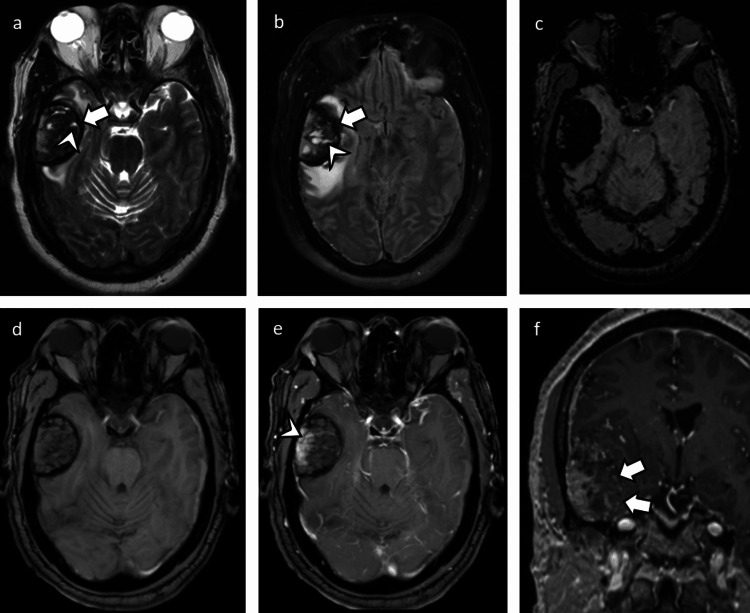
MRI brain without and with contrast a and b: T2 and FLAIR sequences demonstrating a lesion with a T2 hypointense rim (arrows) with surrounding edema. Internal fluid-fluid levels likely related to the internal hemorrhagic component. Additional regions of hemorrhage with fluid-fluid levels (arrowheads) are seen outside of the T2 hypointense rim. c: SWI sequence demonstrating marked susceptibility artifact representative of the hemorrhage, which appears to coincide with the regions that exhibit fluid-fluid levels. d and e: T1 precontrast and postcontrast imaging demonstrate an enhancing component of tumor (arrowhead) along the lateral margin. f: Zoomed-in coronal T1 postcontrast demonstrating enhancing component of the tumor along the lateral margin with the additional suggestion of thin peripheral enhancement (arrows) MRI: magnetic resonance imaging; FLAIR: fluid-attenuated inversion recovery; SWI: susceptibility-weighted imaging
